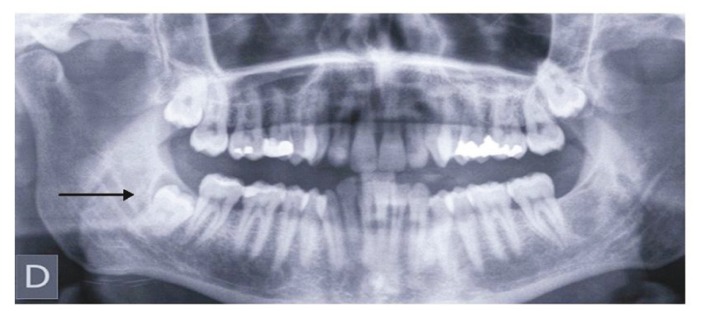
Panoramic radiograph showing an impacted lower third molar completely covered in bone, the tooth indicated for extraction in the case described in the survey.
